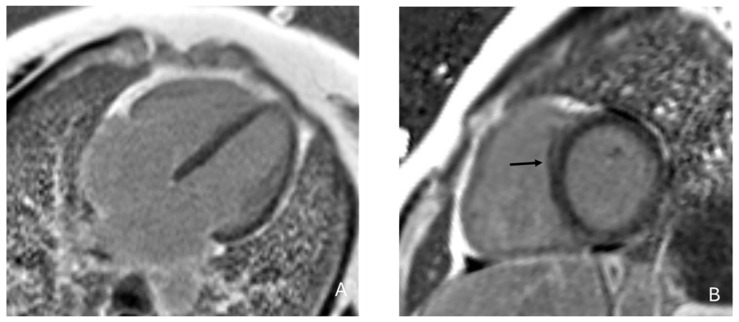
MRI inversion-recovery sequence in four chambers (A) and basal short axis (B). The black arrow shows late enhancement mid-wall at the septum.
